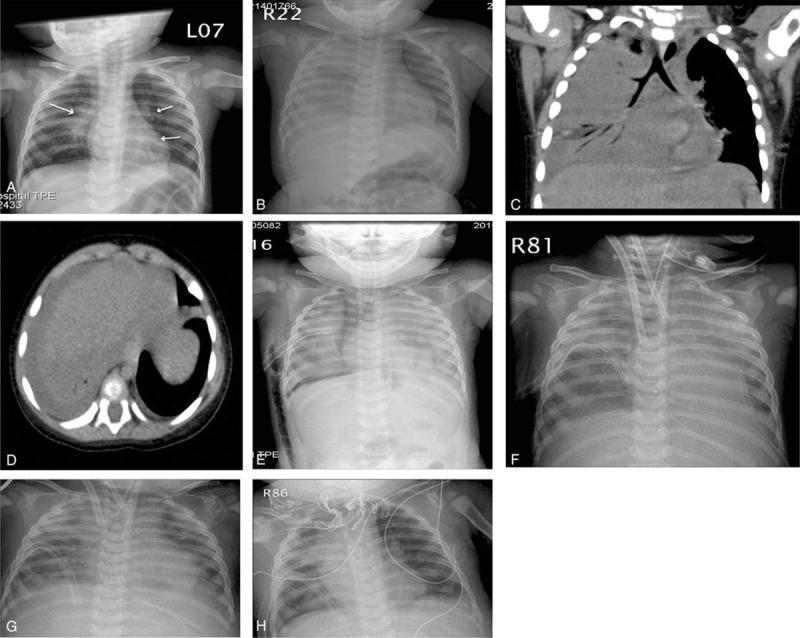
Changes in lung conditions observed on chest x-rays and computed tomography (CT) scans. (A) Chest x-ray on hospital day 1 showed increased bilateral perihilar infiltrations. (B) Chest x-ray on hospital day 5 showed increased infiltration in the left lung field and whiteout in the right lung field with effusion. (C) CT images on hospital day 5 revealed diffuse infiltration in the right lung field with partial collapse and pleural effusion. (D) Sagittal view of chest CT image showing the same findings as (C). (E) Chest x-ray on hospital day 11 showed a chest tube inserted over the right side and progressively increasing infiltrations in bilateral lung fields. (F) Chest x-ray on hospital day 11 after introducing venoarterial ECMO combined with HFOV. (G) Chest x-ray on hospital day 17 after introducing venovenous ECMO revealed improvements in bilateral lung expansion while under EMCO and HFOV. (H) Chest x-ray on hospital day 20 after removing ECMO showed better lung recruitment with reduced bilateral infiltrations. ECMO = extracorporeal membrane oxygenation, HFOV = high-frequency oscillatory ventilation.
